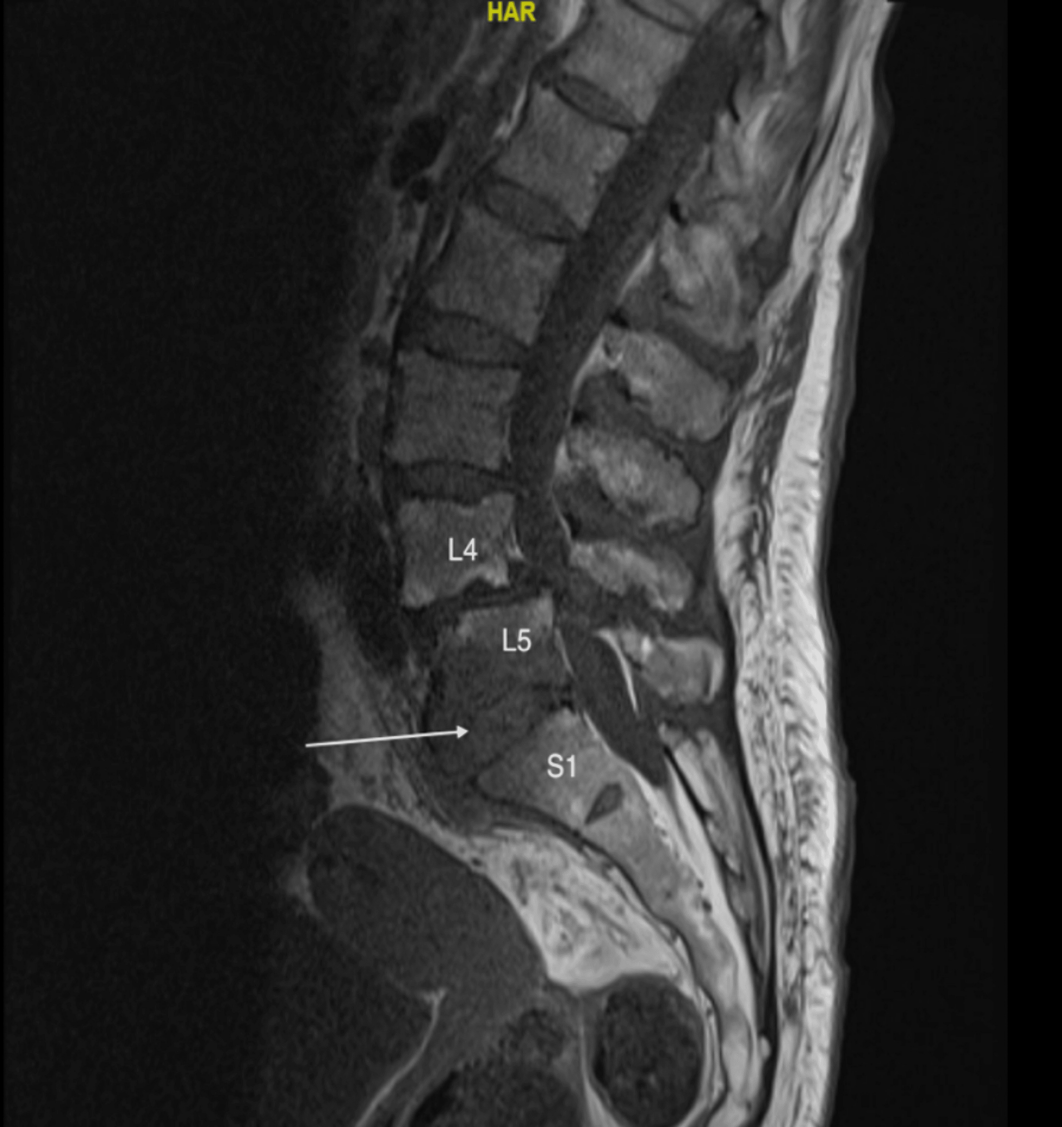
An MRI of the lumbar spine showed circumferential soft tissue fullness with irregularities and erosive findings of the superior and inferior endplates, consistent with discitis at the L5-S1 level (white arrow) MRI: magnetic resonance imaging
